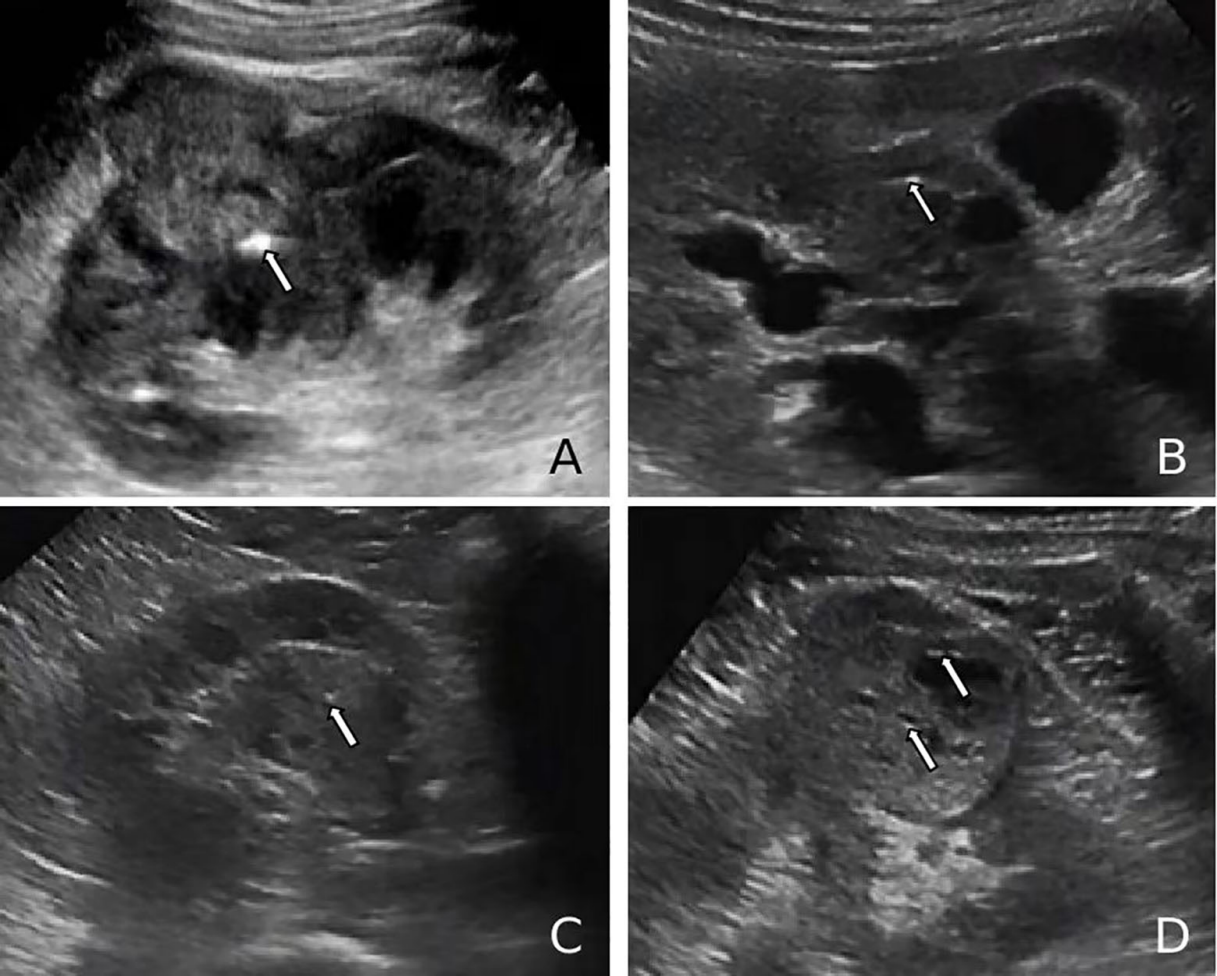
(A, B) Ultrasound images of renal cell carcinoma (RCC) demonstrating a markedly hyperechoic intratumoral lesion (arrows), exceeding the echogenicity of the renal sinus, consistent with a calcification focus. (C, D) Ultrasound images of RCC showing punctate hyperechoic foci within the lesion and anechoic areas peripherally (arrows), suggestive of posterior acoustic enhancement and calcified cyst wall.
